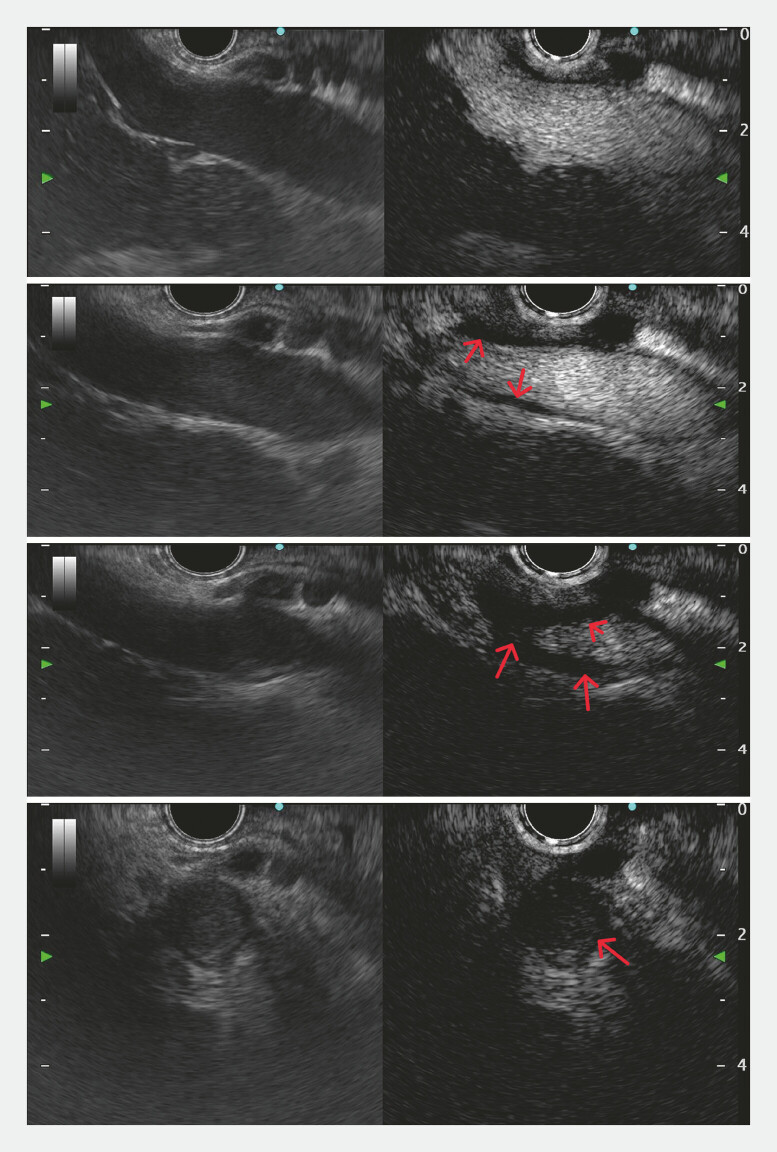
CEUS showed the demarcated thrombotic areas without internal enhancement from the non-thrombotic area (red arrow). CEUS, contrast-enhanced endoscopic ultrasound.
